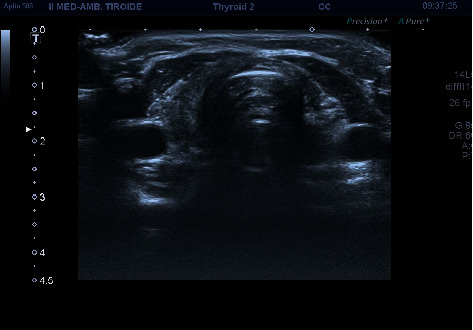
Thyroid ultrasound revealed an atrophic gland without nodules.
